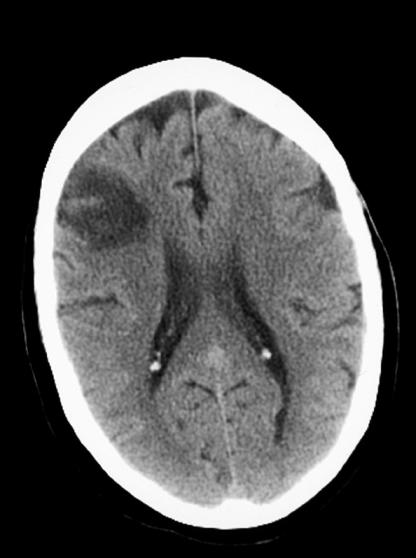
CT Brain Scan on Admission Showing an Infarct in the Posterior Right Frontal Lobe
